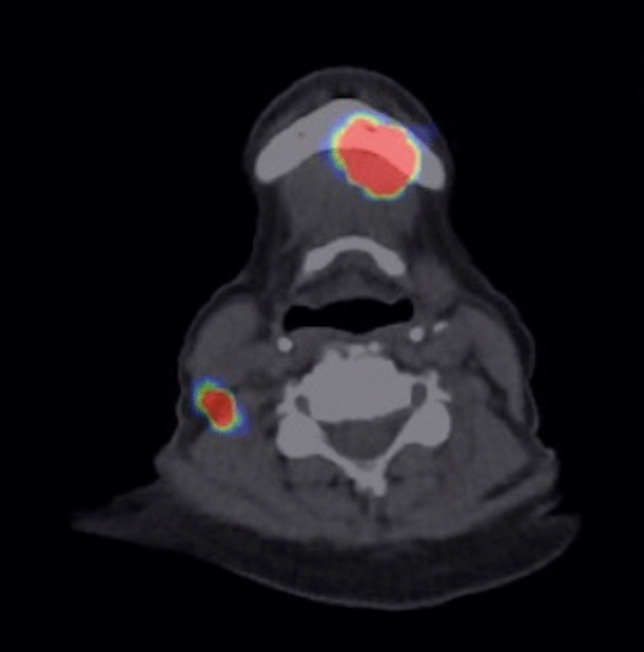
Visability of the primary and the SLN in the preoperative SPECT/CT after injection of nanosized 99mTc colloid
